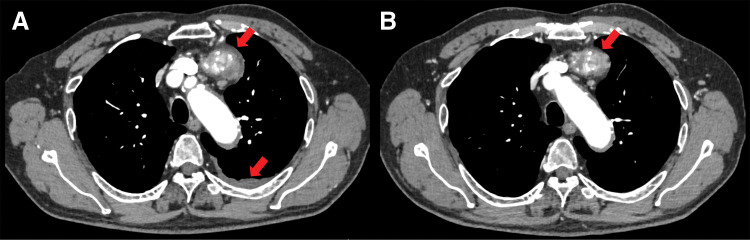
Case 3. Finding of chest CT. (A) Chest CT showed a 74 × 66 × 58 mm tumor with internal low-density area and contrast enhancement at the margins in the anterior mediastinum (arrows). A small amount of pleural effusion was observed on the left side. (B) Chest CT about 2 months after the first presentation revealed tumor regression (arrow) and disappearance of the pleural effusion.
